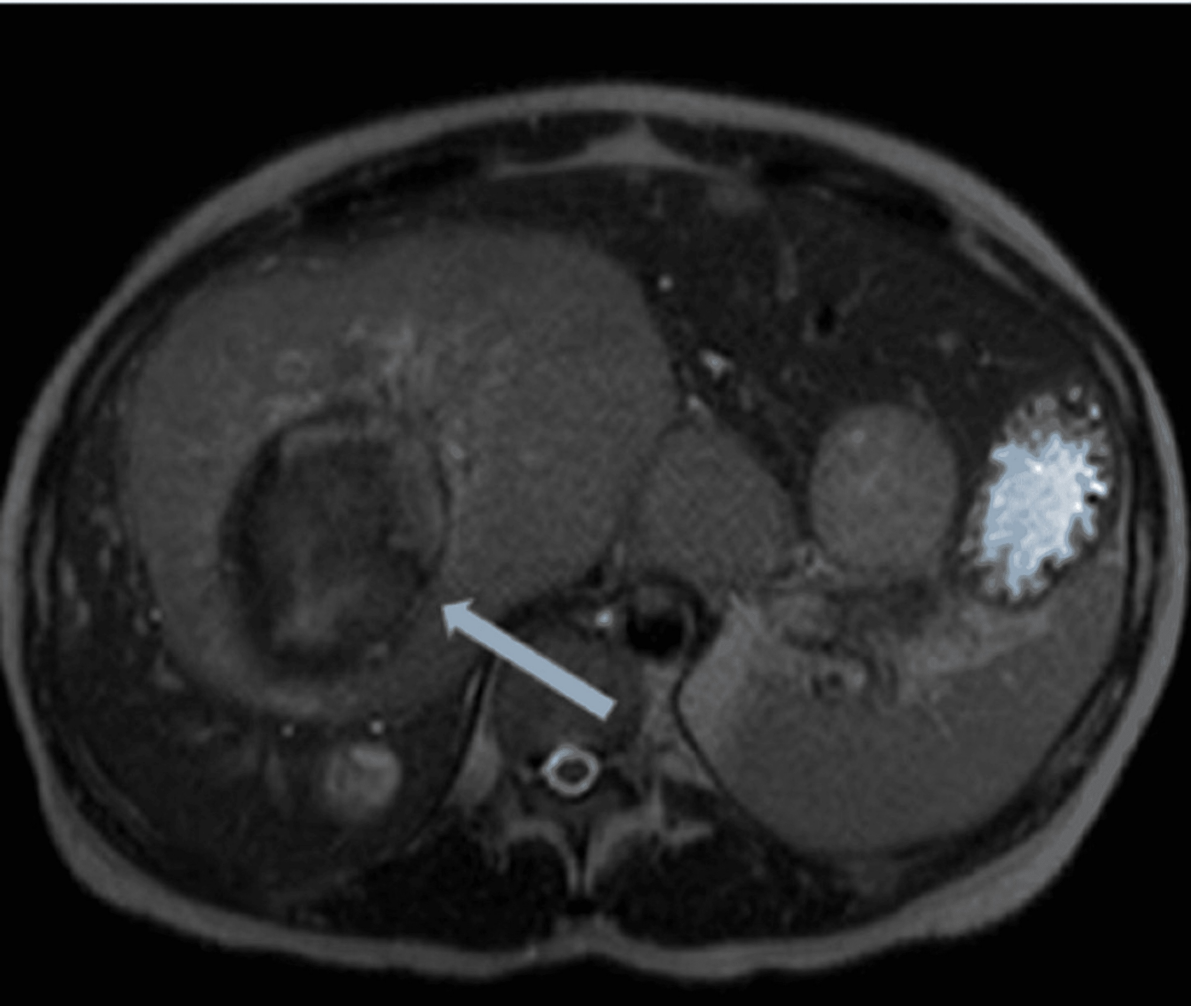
MRI of the Liver With/Without IV Contrast Liver Mass: 10.5 x 13.5 x 13.1 cm of segment 4/8, partially necrotic
